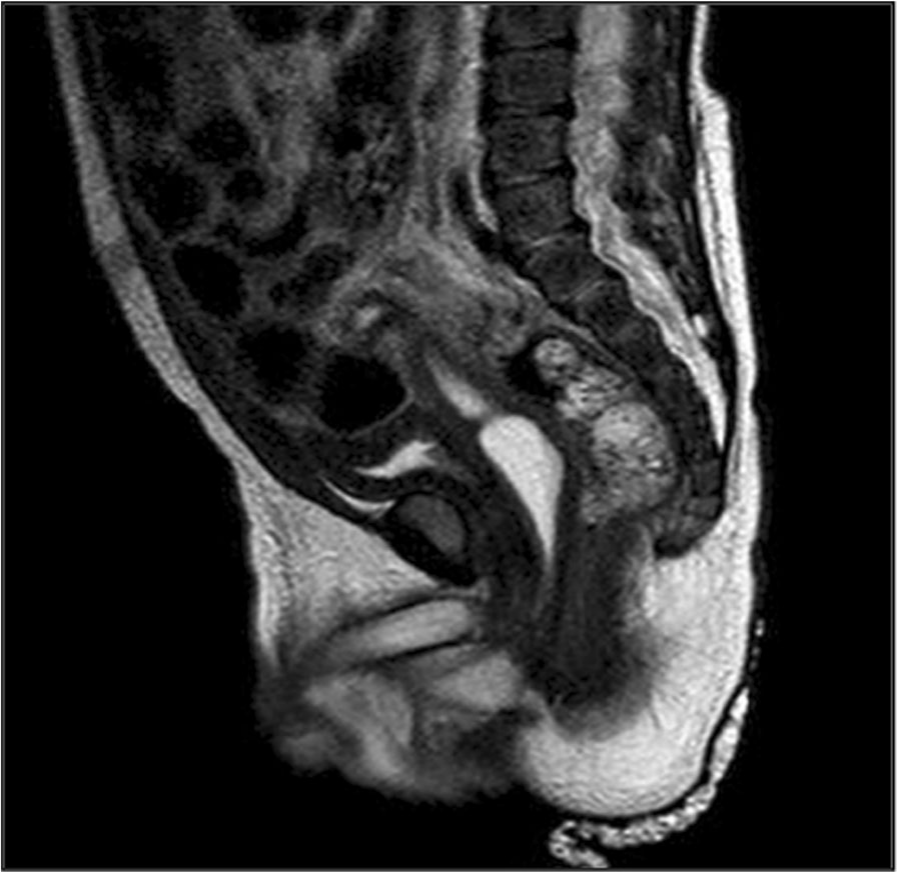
X-ray saggital image of MRI of case 1
